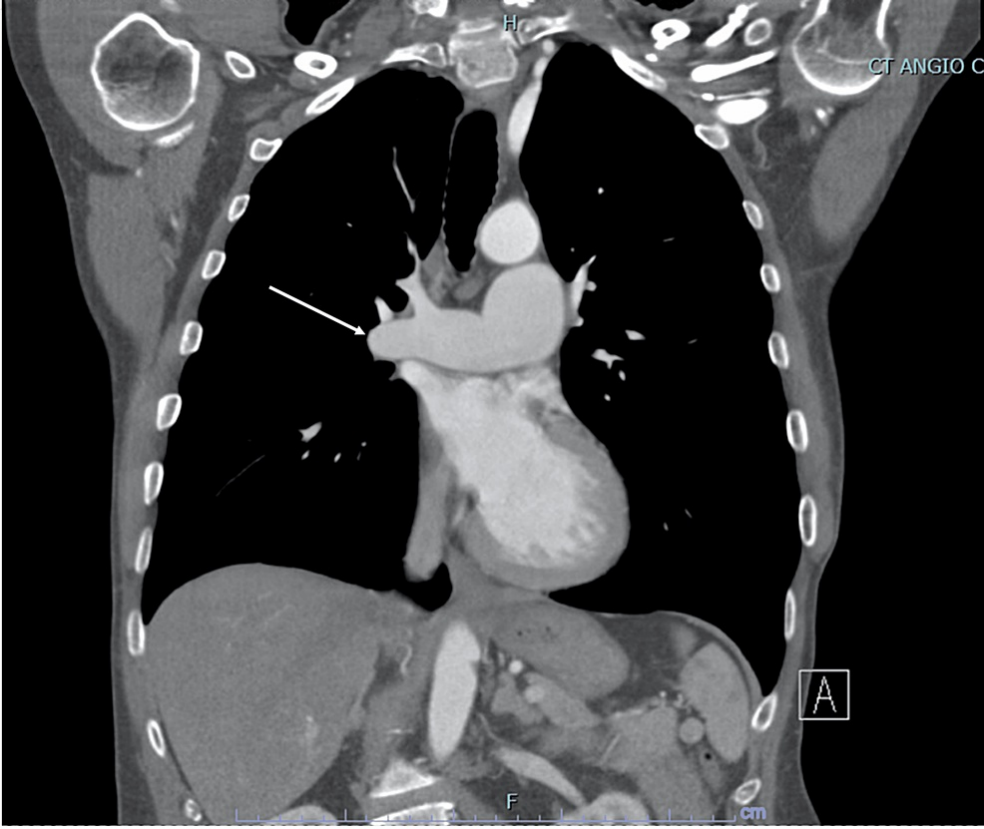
CTA chest identifying marked enlargement of the main central pulmonary arteries. CTA, computed tomography angiography
